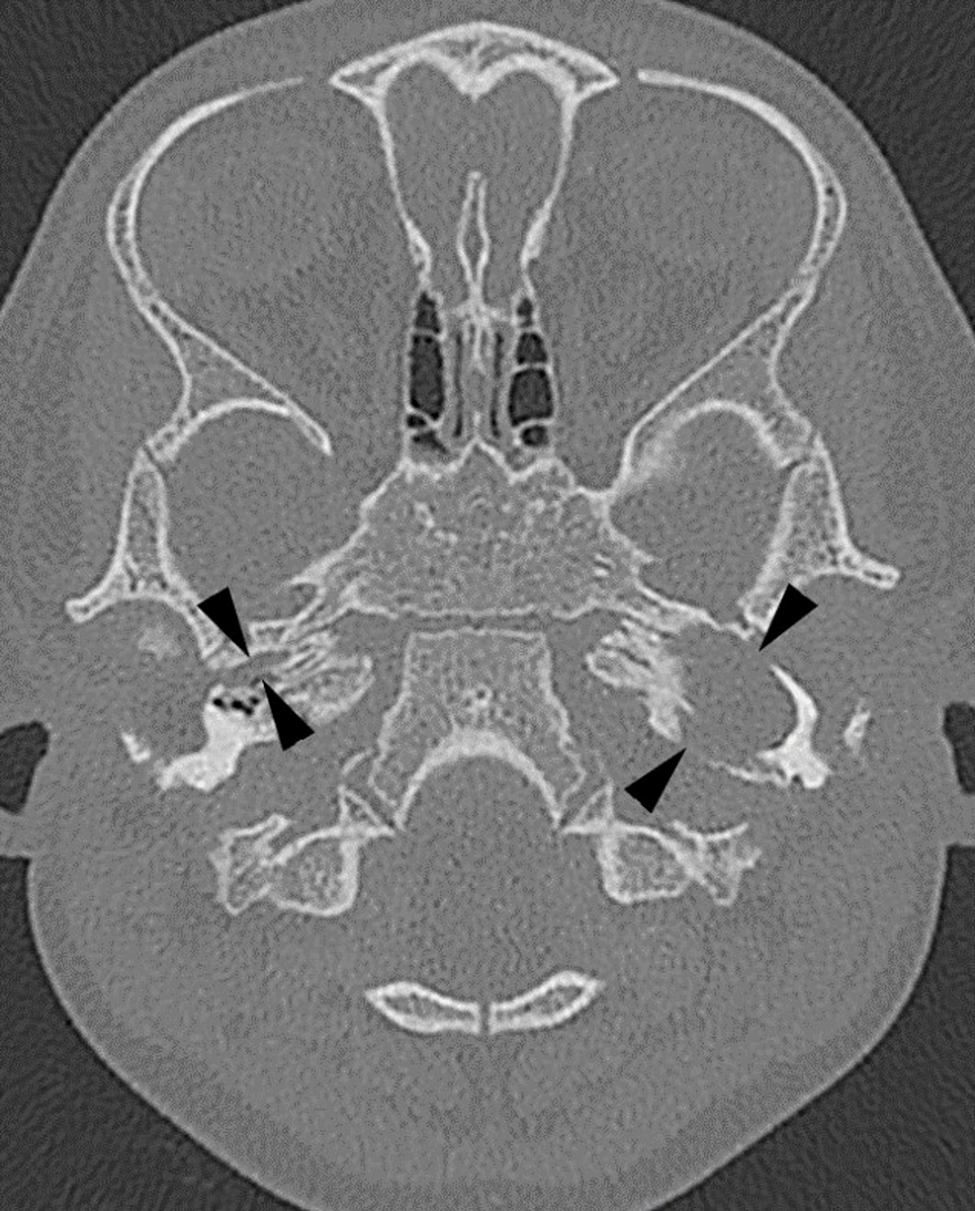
Axial HRCT temporal (bone algorithm) Axial cut at the most superior aspect of the fatty lesion displays widening of the osseous left Eustachian tube [note the normal osseous Eustachian tube on the right (black arrowheads)] HRCT: high-resolution computed tomography
